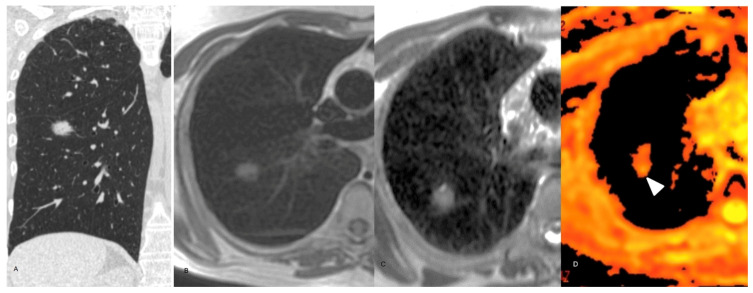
62-year-old man staging colon cancer. (A) Coronal reconstruction non-enhanced CT chest, lung window, showing a spiculated 8 mm nodule in the superior segment of the right lower lobe. (B) Axial T1-weighted non-enhanced chest MRI demonstrating the same nodule. (C) Axial T2-weighted chest MRI. The nodule exhibits high signal in both sequences. (D) DWI axial chest MRI showing marked diffusion restriction (arrow head). The final diagnosis was metastatic adenocarcinoma with lepidic growth.
